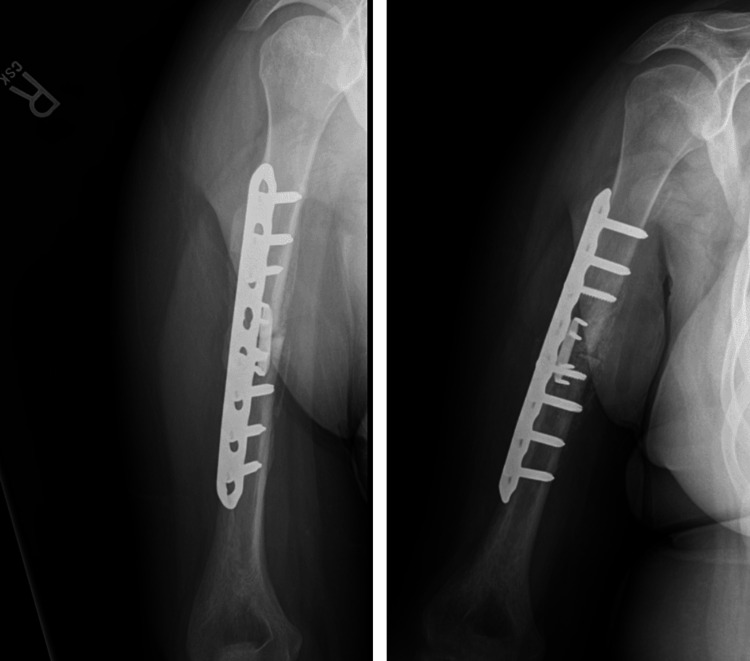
X-rays of the right humerus at a three-month post-operative visit. A healing reaction surrounding the bone is noted extending proximal and distal to the fracture site.
